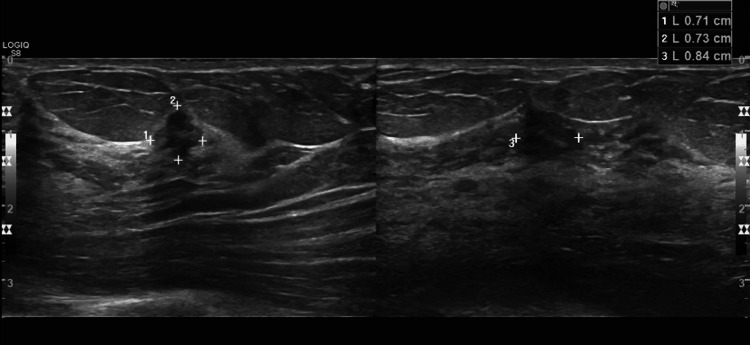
Ultrasound of the 10 o'clock mass Ultrasound findings stated that "The 0.7 cm x 0.7 cm x 0.8 cm mass in the right breast at 10 o'clock middle depth appears suspicious of malignancy."
